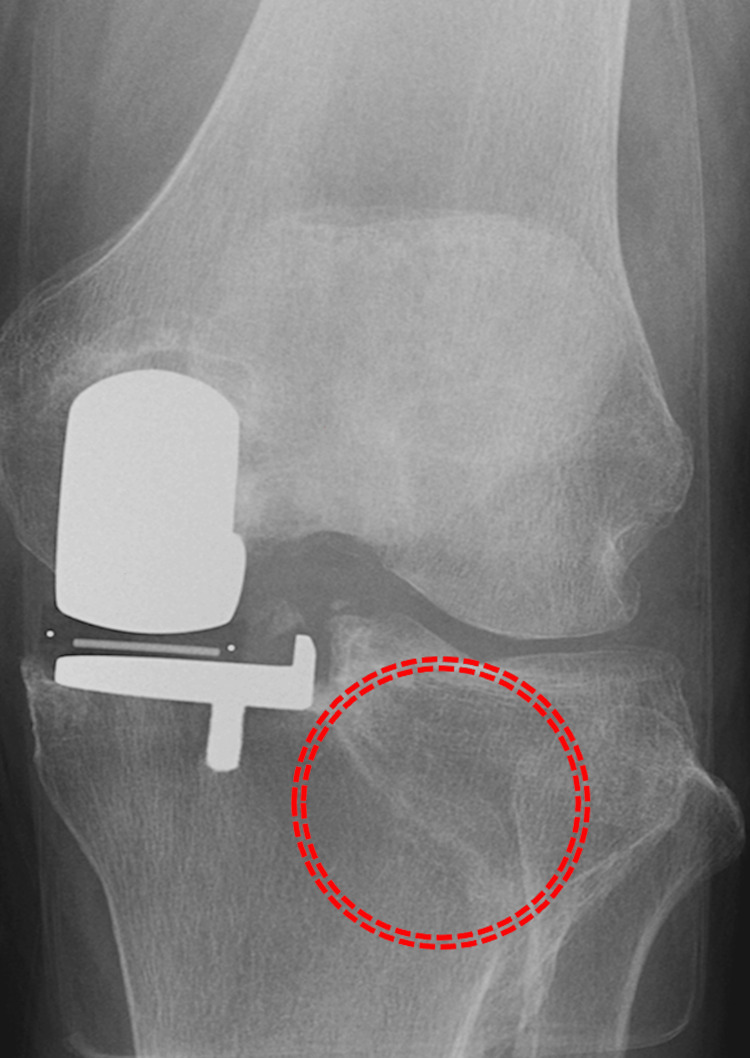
No obvious displacement is observed on the X-ray one year after surgery (dotted circle).
